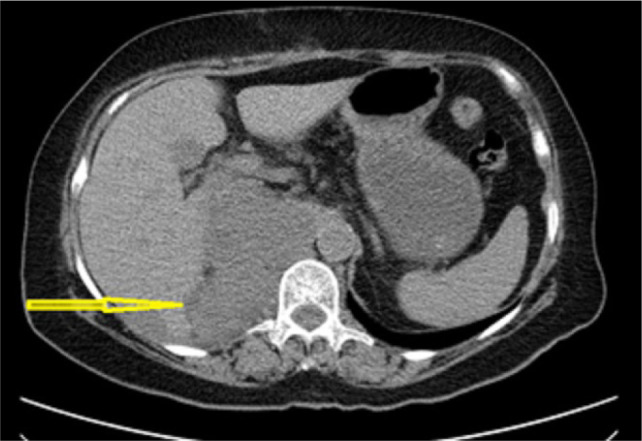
Postoperative abdominopelvic CT scan demonstrates local recurrence and liver metastasis (Arrow).
